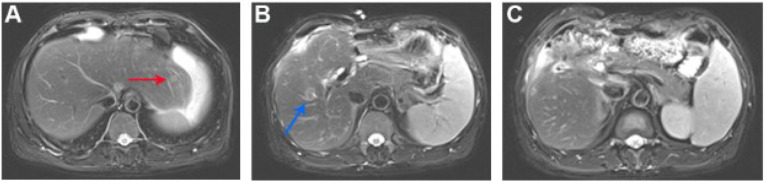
After five cycles of combinative treatment, MRI of the patient liver showed that liver metastasis sites diminished or disappeared. (A) The metastatic nodule in the left outer upper segment of the liver diminished (the red arrow showed the position of the previous nodule; Figure 3A); (B) The metastatic nodule in the center of the right upper liver diminished (the blue arrow showed the position of the previous nodule; Figure 3B); (C) The metastatic nodules under the capsule disappeared (Figure 3C).
